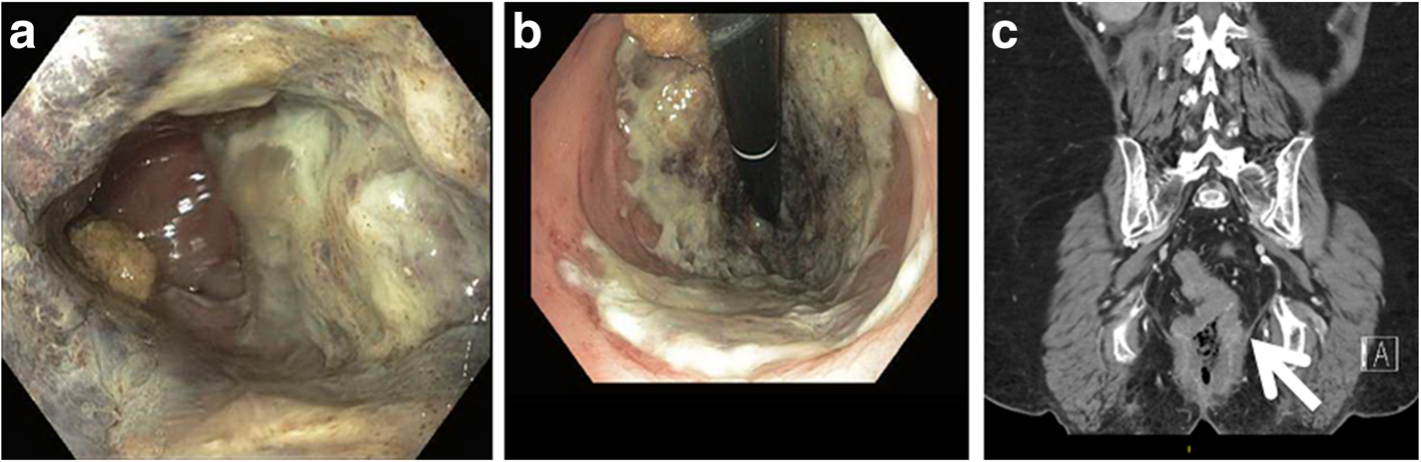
a and b Rectal mucosa with ulcerations, exudates, and purplish hue. c CT scan showing circumferential rectal wall thickening (arrow) with adjacent fat stranding and apparent adhesion to the mesorectal fascia
